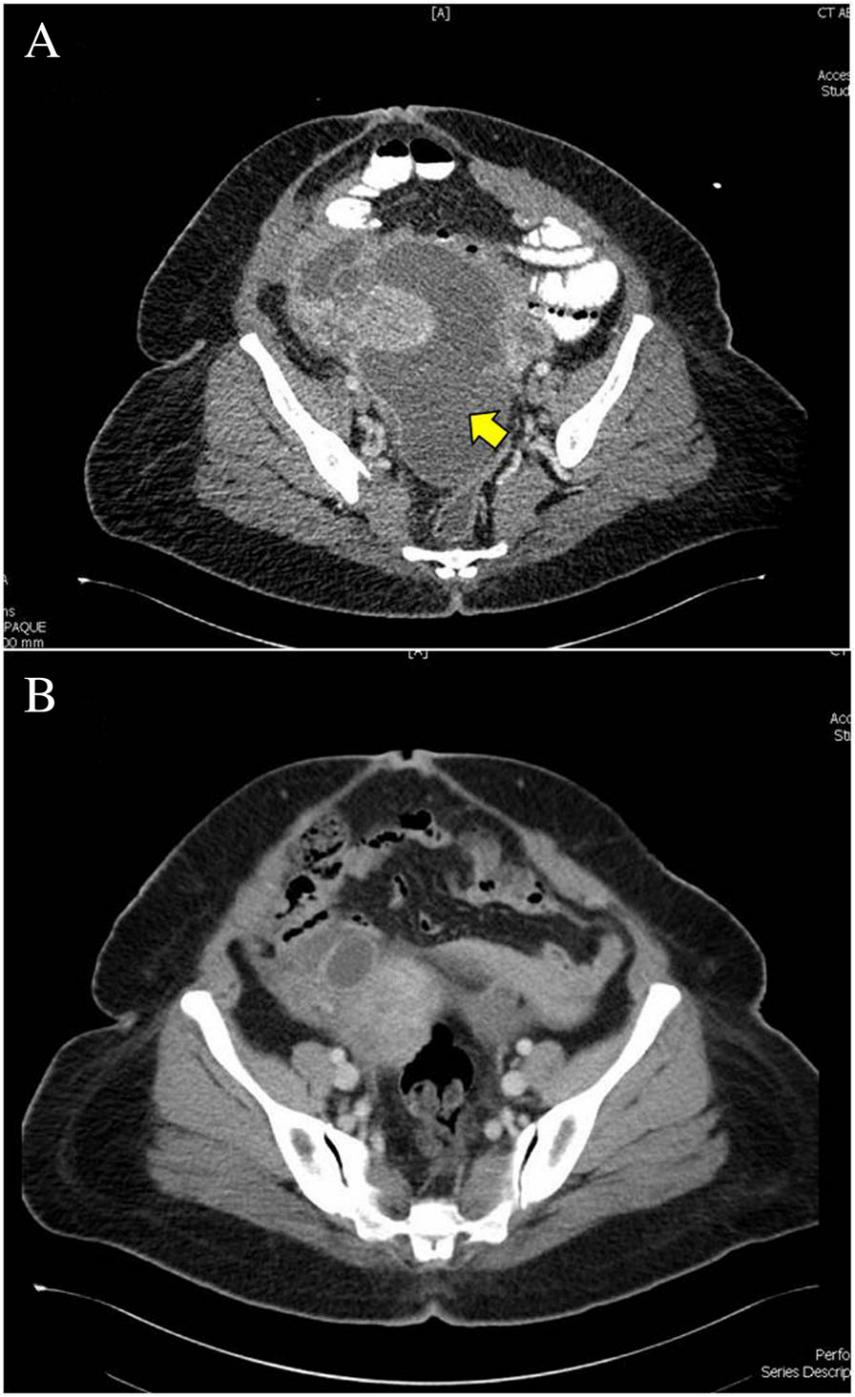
A 44-year-old woman presented with abdominal pain of 3 days duration. The abdomen was distended, tender, but soft. The patients had fever, leukocytosis, and raised C-reactive protein. Abdominal CT scan (a) showed multiple intra-abdominal fluid collections (yellow arrow). Green pus was aspirated under ultrasound guidance. The pus culture was negative, and the quantiferon-TB test was undetermined. Abdominal tuberculosis was suspected. A therapeutic diagnosis was successful and the size of the abscess dramatically reduced after 2 months (b). (Courtesy of Dr. Hussam Mousa, Consultant General Surgeon, Al-Ain Hospital, Al-Ain, UAE)
